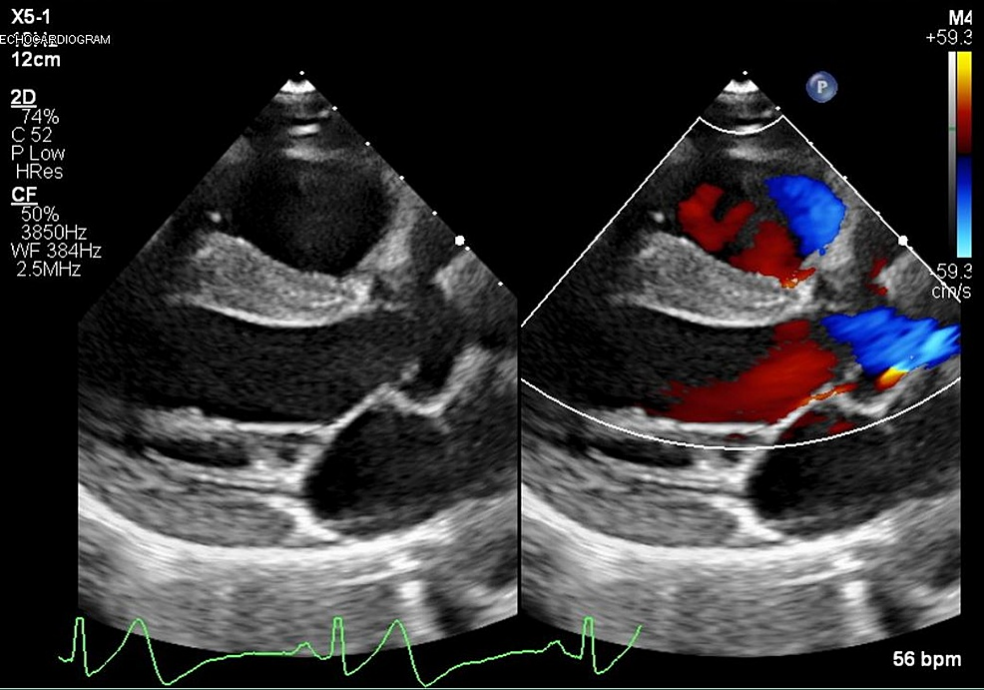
TTE-modified parasternal long-axis view demonstrating a dilated aortic root with giant right coronary artery aneurysm TTE, Transthoracic echocardiography.
